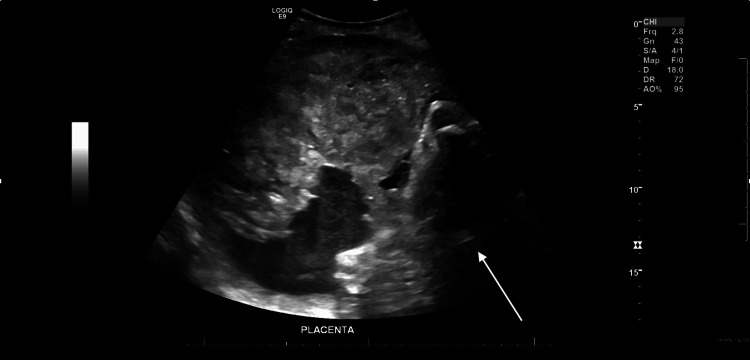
Ultrasonographic visualization demonstrating placental separation from the uterine wall (white arrow), consistent with abruption.
